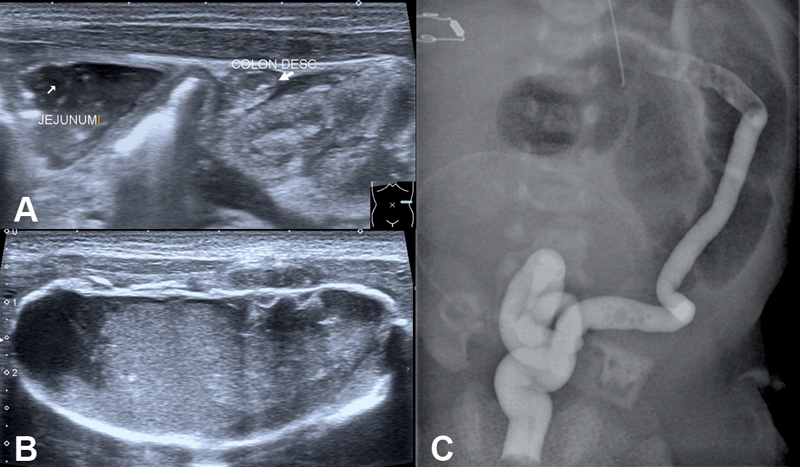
Ultrasound and contrast enema. (
